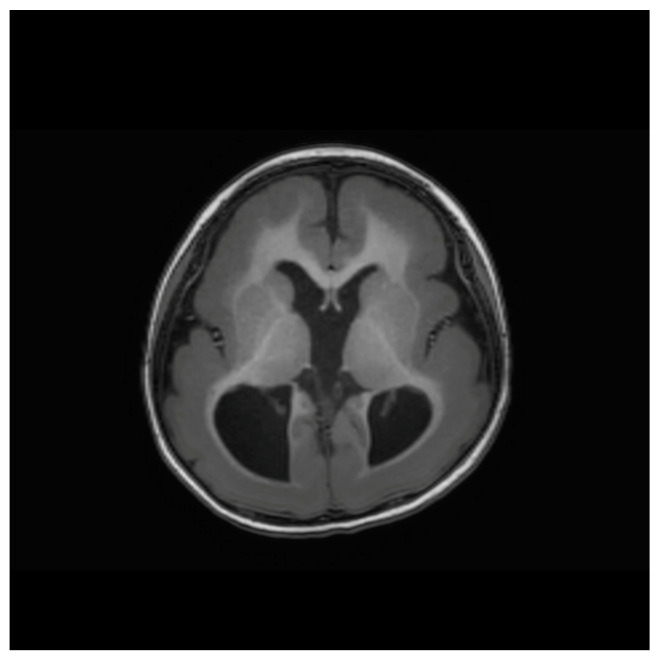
Brain magnetic resonance image (MRI) showing lissencephaly.
